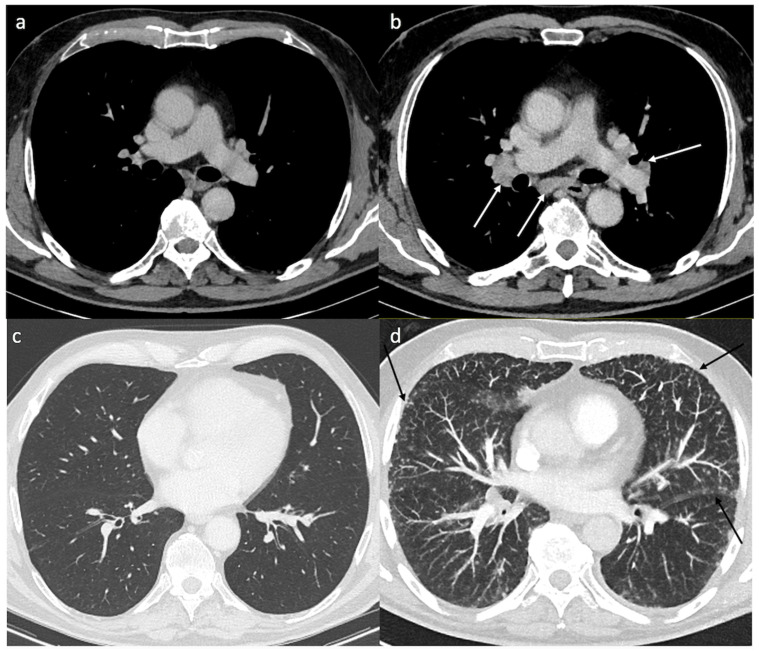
Typical findings of pulmonary sarcoidosis. Axial contrast enhanced computed tomography (CT) shows no mediastinal lymph node enlargement (a) and no parenchymal disease (c) before treatment. Five months after immunotherapy, axial enhanced CT shows typical bilateral and symmetric hilar and subcarinal lymph nodes (b) and multiple micronodules with a perilymphatic distribution (subpleural and perifissural nodules) (black arrows-d).
